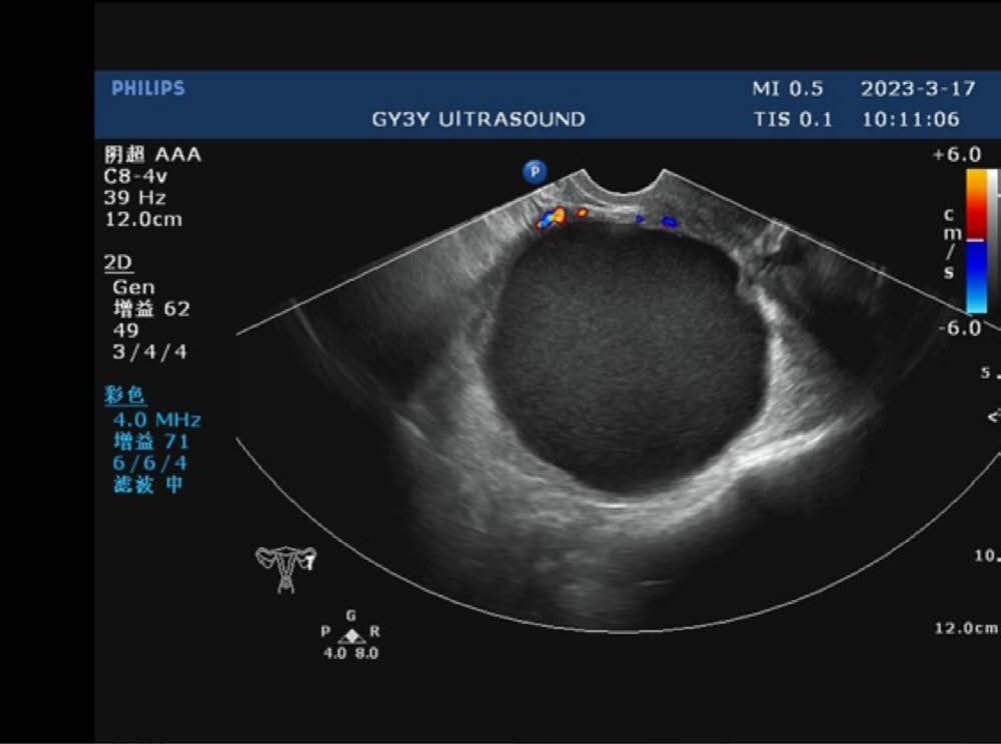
Routine gynecological ultrasound examination revealed a round-shaped hypoechoic area in the left ovary, measuring 81 × 74 mm, with clear borders and filled with fine dot-like low echoes.
